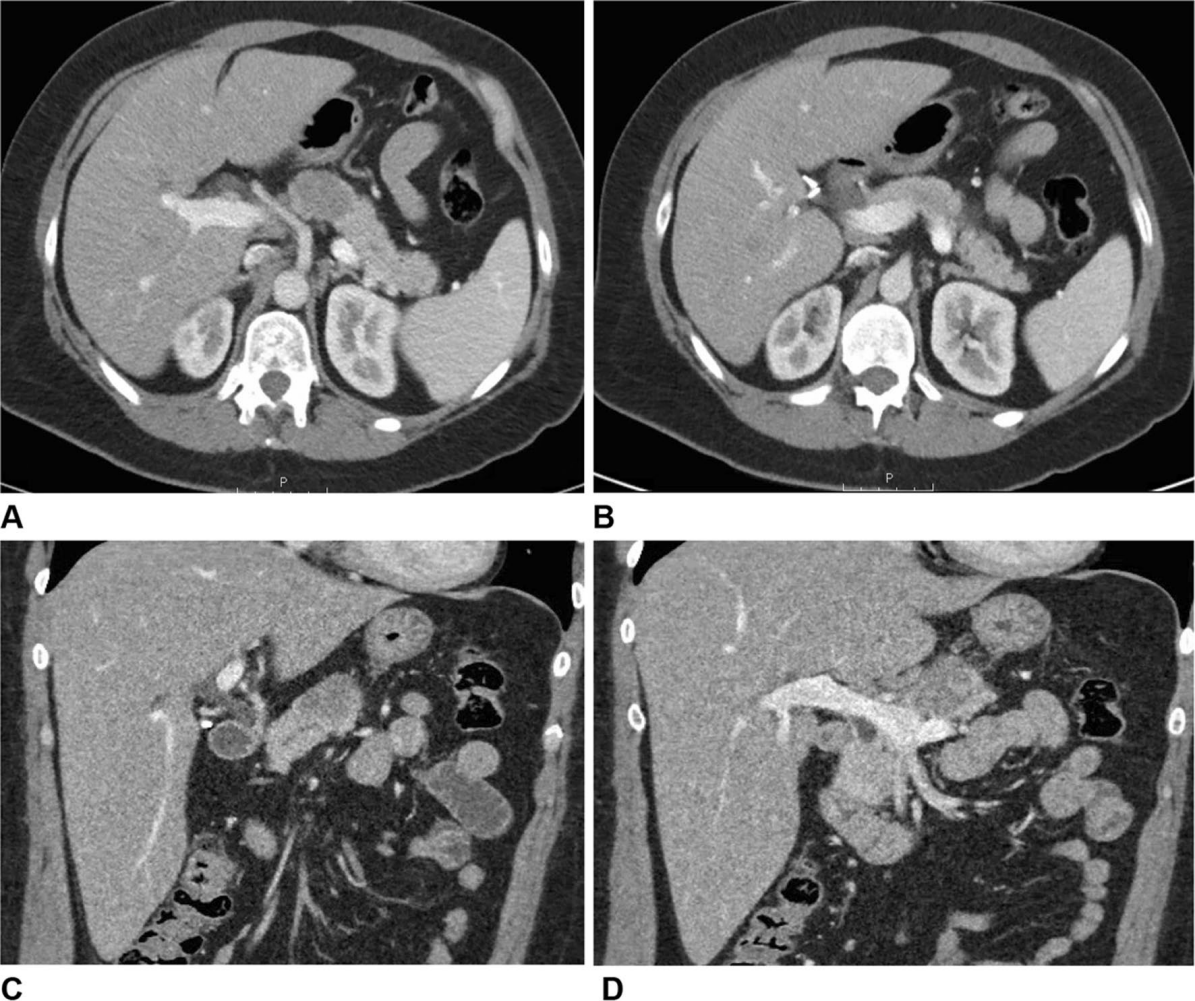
CT scan abdomen/pelvis with and without contrast. Axial (A, B) and coronal (C, D) views demonstrating pancreatic mass
